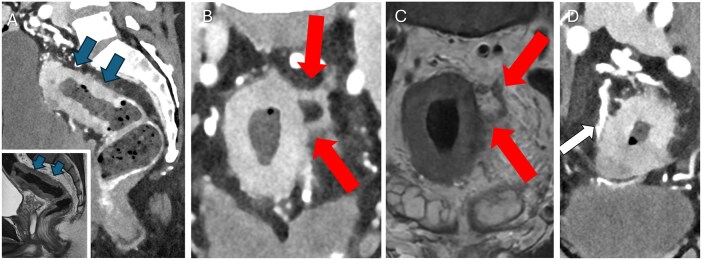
A 58-year-old male with mid-rectal cancer demonstrated on sagittal image from bolus-tracked, portal phase PCD-CT (A, blue arrows; corresponding MRI, inset). Coronal 40-keV PCD-CT image shows the circumferential cancer with a thick, enhancing wall and tumor extending into small left superior pararectal veins representing extramural venous invasion (B, red arrows), also shown on rectal cancer MRI later the same day (C, red arrows). Note that on portal phase 40-keV PCD-CT images arteries and veins are brightly opacified and appear white (D, white arrow) on another coronal image. Extramural venous invasion is an imaging feature of advanced rectal cancer and is associated with distant metastases, higher rates of local recurrence, and decreased survival. Abbreviation: PCD, photon-counting detector.
